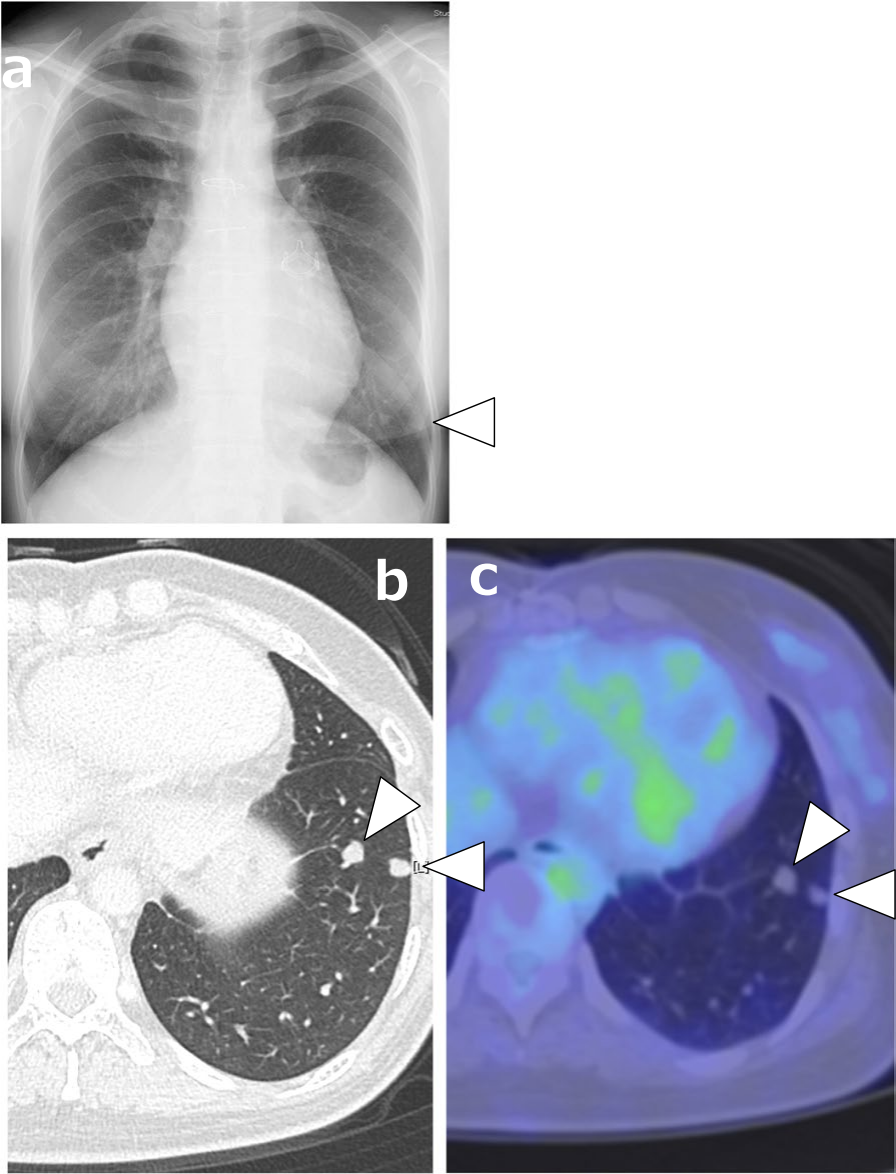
a Chest X-ray findings: small nodular shadow (arrowhead) was observed in the left lower lung field. b Chest computed tomography findings: two well-demarcated solid nodules (arrowheads) were observed in the segment 8 region of the left lower lobe, 9 mm and 7 mm in diameter. c^18^F-fluorodeoxyglucose positron emission tomography (FDG-PET) findings: no accumulation was observed in pulmonary nodules (arrowheads) or other areas
